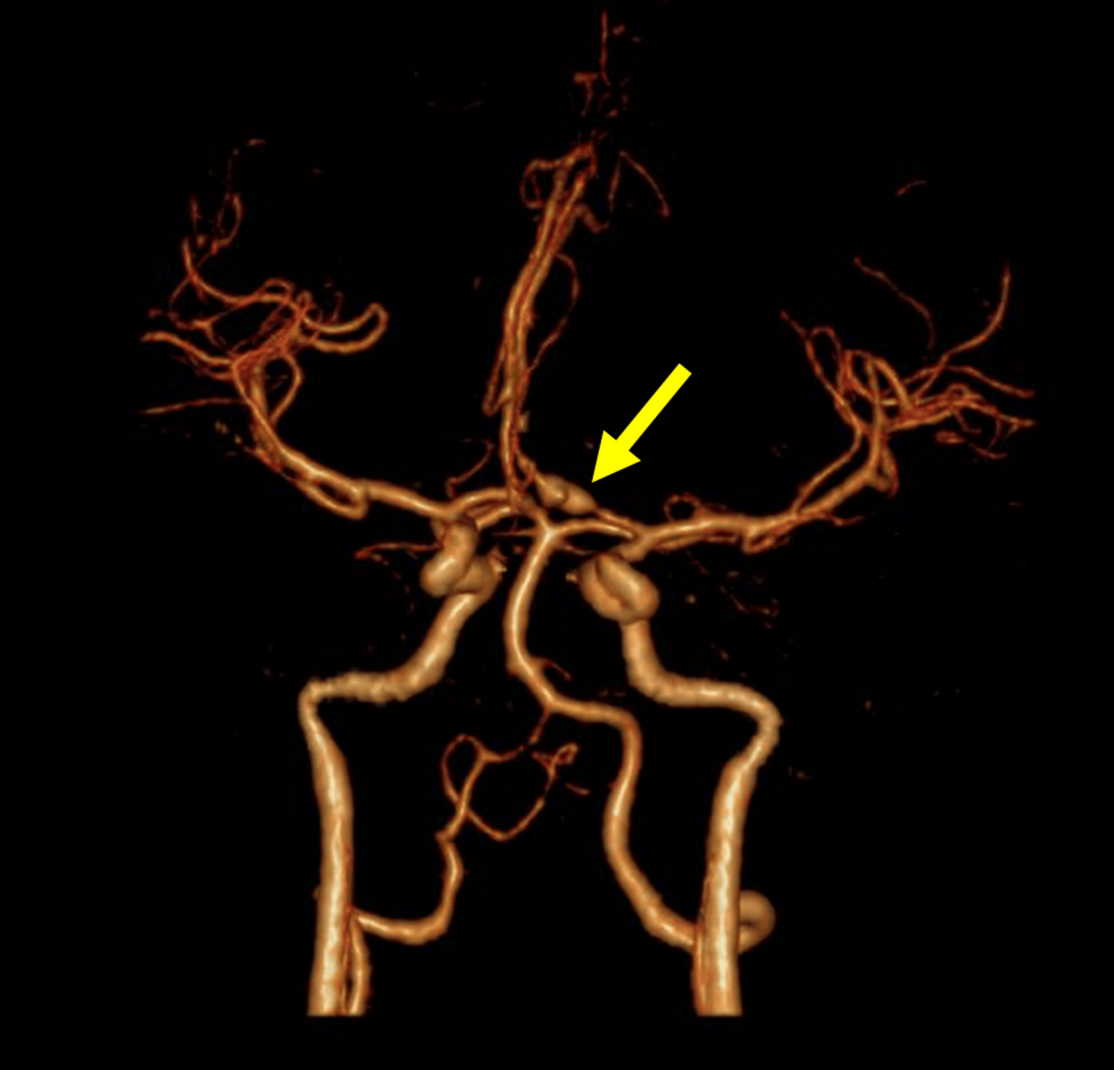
Cranial CT angiogram CT: computed tomography
